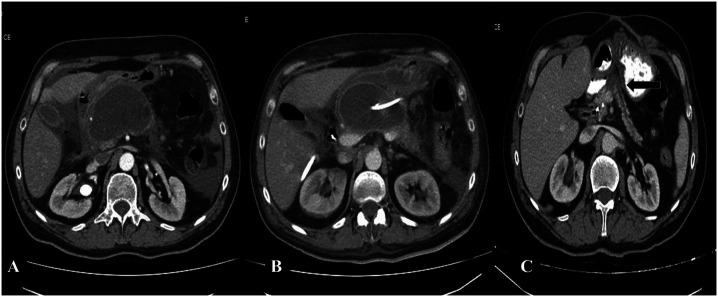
A 57-year-old male patient. A) A 10 cm-sized WON in the pancreatic head-body segment. B) Initial percutaneous intervention due to abscess symptoms abscess symptoms. C) Surgical necrosectomy was performed after 2 weeks because of no response to percutaneous intervention. Patient developed cystocutaneous fistula with atrophy and dilated duct in the pancreatic tail seen on CT imaging (disconnected pancreatic duct) (Black arrow).
